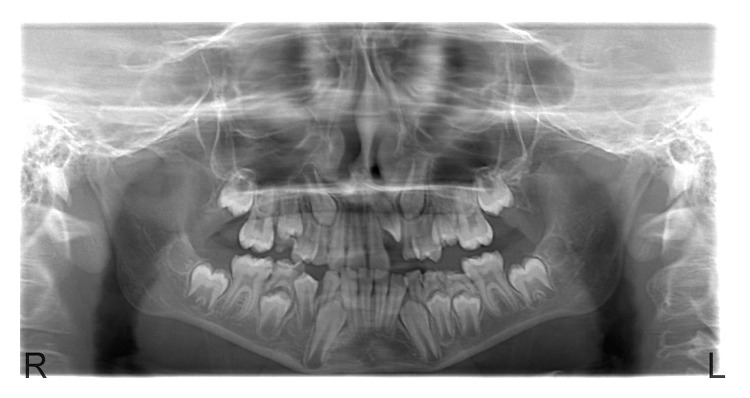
Orthopantomographic view after trauma.
